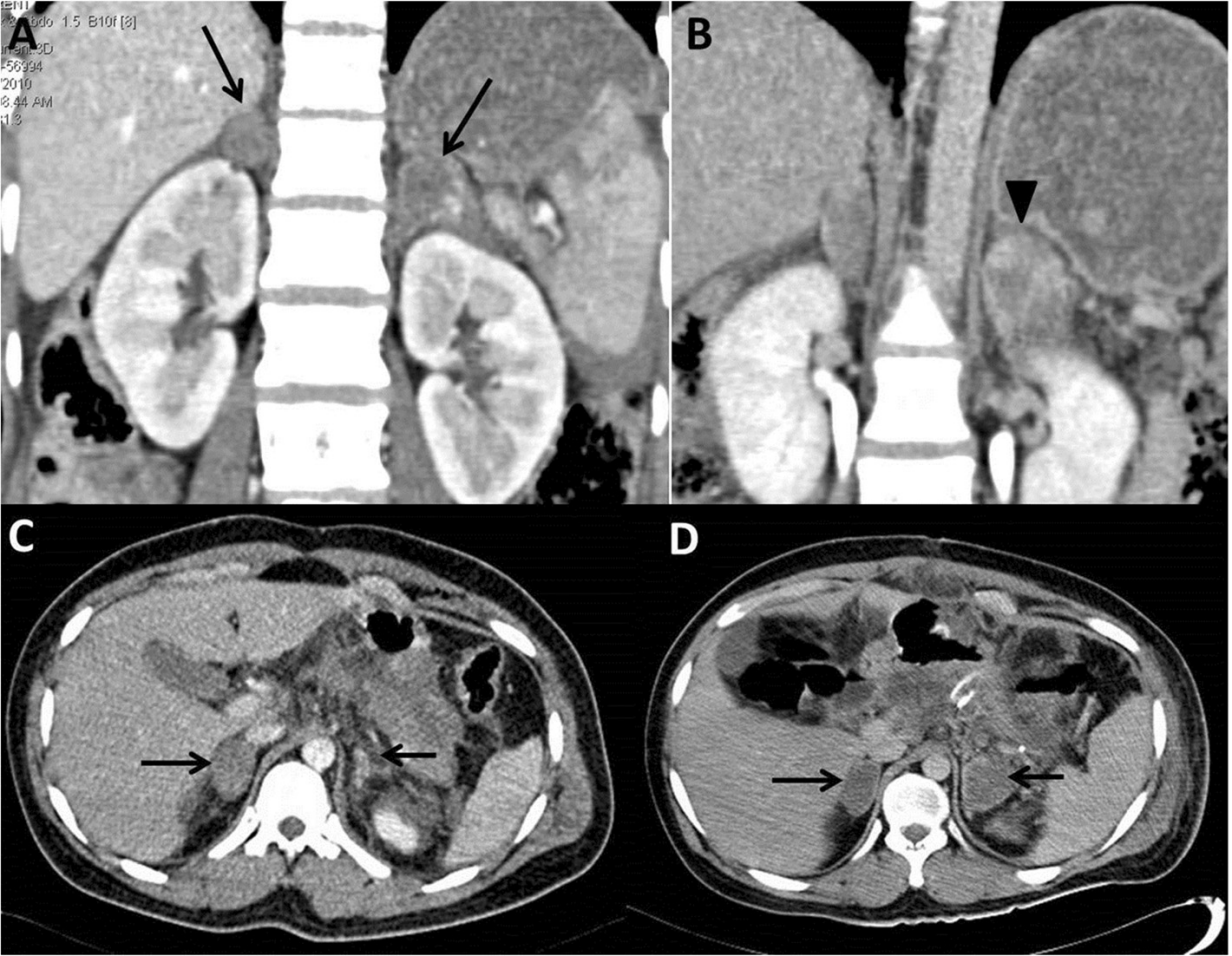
Bilateral adrenal injuries. CT coronal sections (a,b) show bilateral adrenal hematomas (arrows, a) with active extravasation of contrast in the left adrenal gland on delayed scan (arrowhead, b). Due to persistent hypotension, intraoperative packing of left adrenal gland with surgigel was done but patient died within 48 h due to intractable hypotension. CT axial sections (c,d) of another patient show right oval hematoma and left bulky gland on day 1 CT (arrows, c). On follow-up day 10 CT, the left adrenal injury evolved into an oval hematoma (arrows, d)
